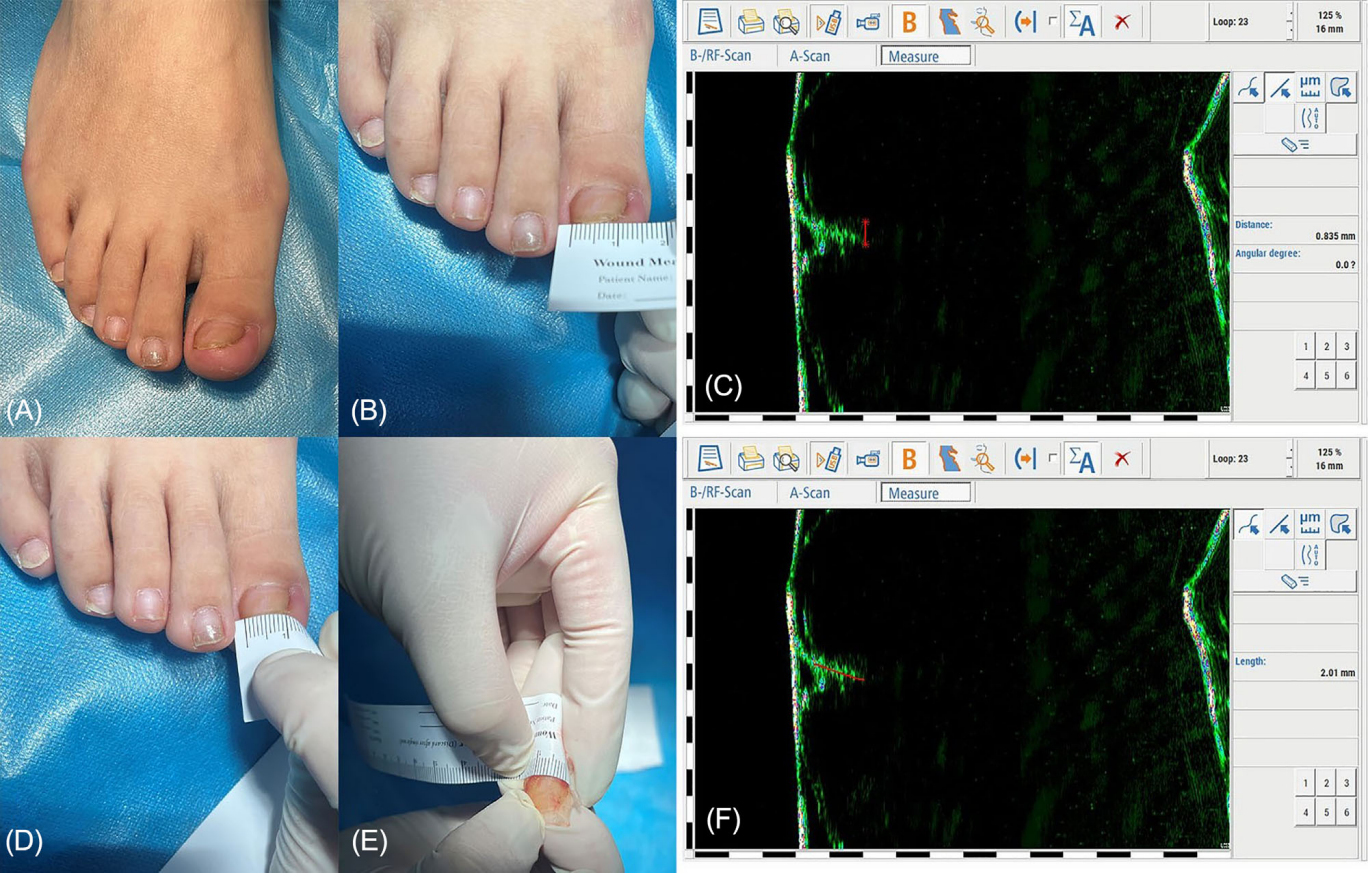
The use of high‐frequency ultrasonography (HF‐USG) complemented the data of the covered nail tip, which were combined with the data obtained from the conventional measurement of the apparent nail plate for an accurate preoperative measurement. (A) The case of the pincer nail with unexposed hypertrophy of the lateral and distal nail folds and both sides of the distal nail tip. (B) The apparent width of the distal nail plate. (C) The width E2 of the covered nail plate complemented by HF‐USG. (D) The length (10 mm) of the distal nail plate measured with the evaluation system of the curvature index. (E) The length (14 mm) of distal the nail tip of the nail plate removed during surgery, clearly inconsistent with the preoperative data of 10 mm. (F) Preoperatively, the length D2 of the nail tip of the covered part complemented using HF‐USG, and D3 was also measured, which were added with D1 data, consistent with the postoperative data.
